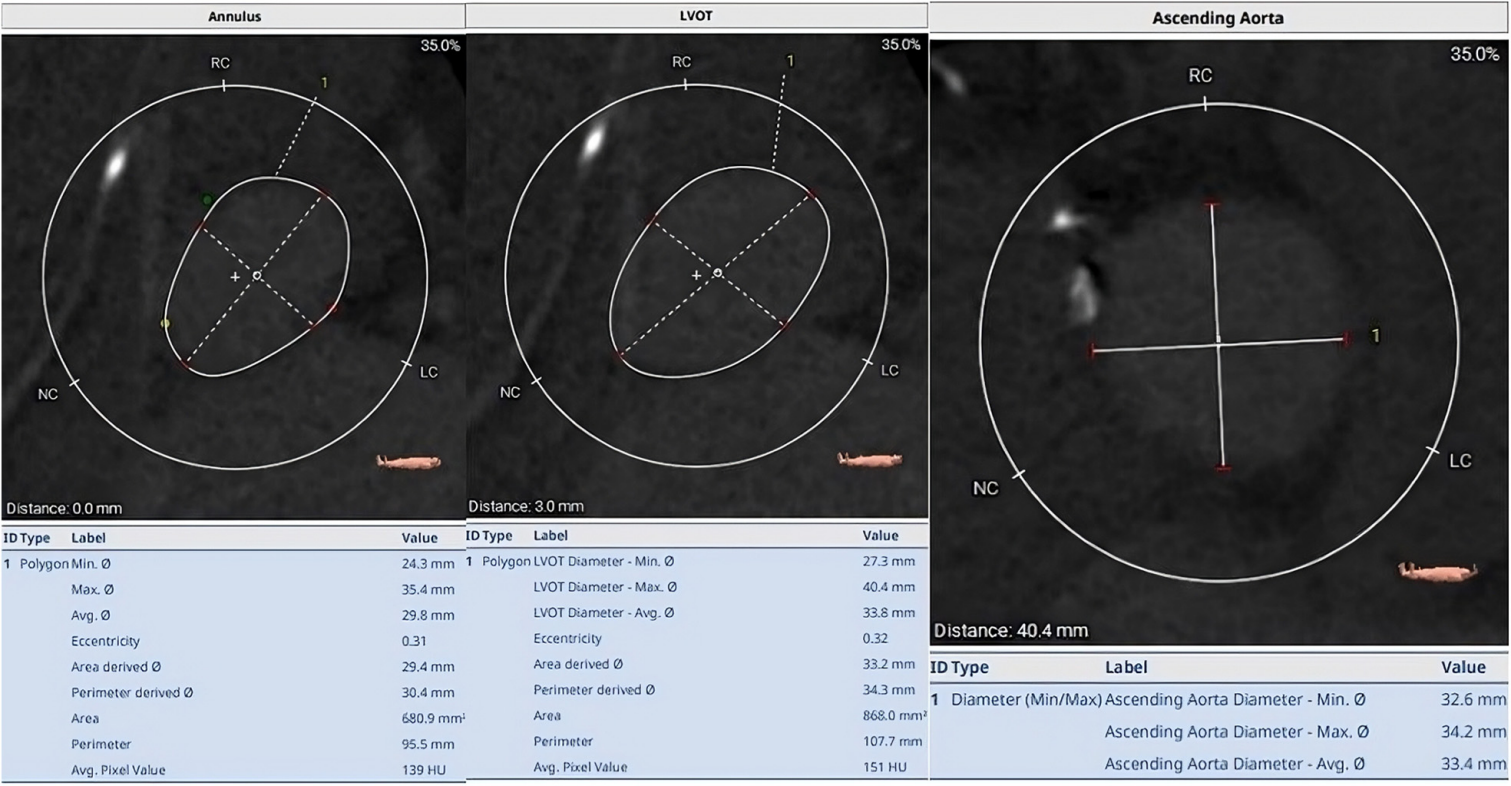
Patient 1: Preprocedural computed tomography. LVOT, left ventricular outflow tract.
